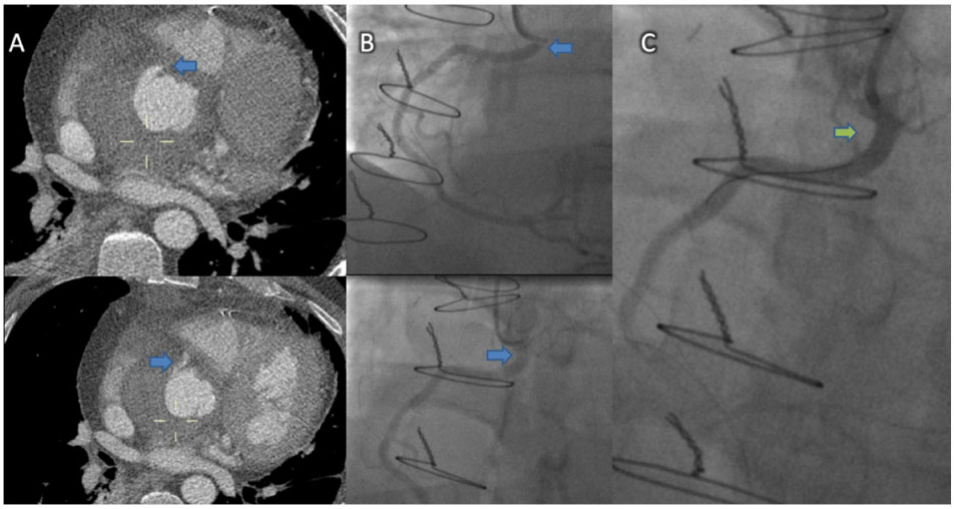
A 53-year-old man with a bicuspid aortic valve underwent elective cardiac surgery involving the David procedure. Four days post-operatively developed non-ST-elevation myocardial infarction. Gated computed tomography angiography suggested distortion of the proximal right coronary artery marked with a blue arrow (*A*). This corresponds with the stenosis marked with blue arrows in diagnostic invasive angiography images (*B*). There was a good result following implantation of a drug-eluting stent with the stented area marked by the green arrow (*C*).
